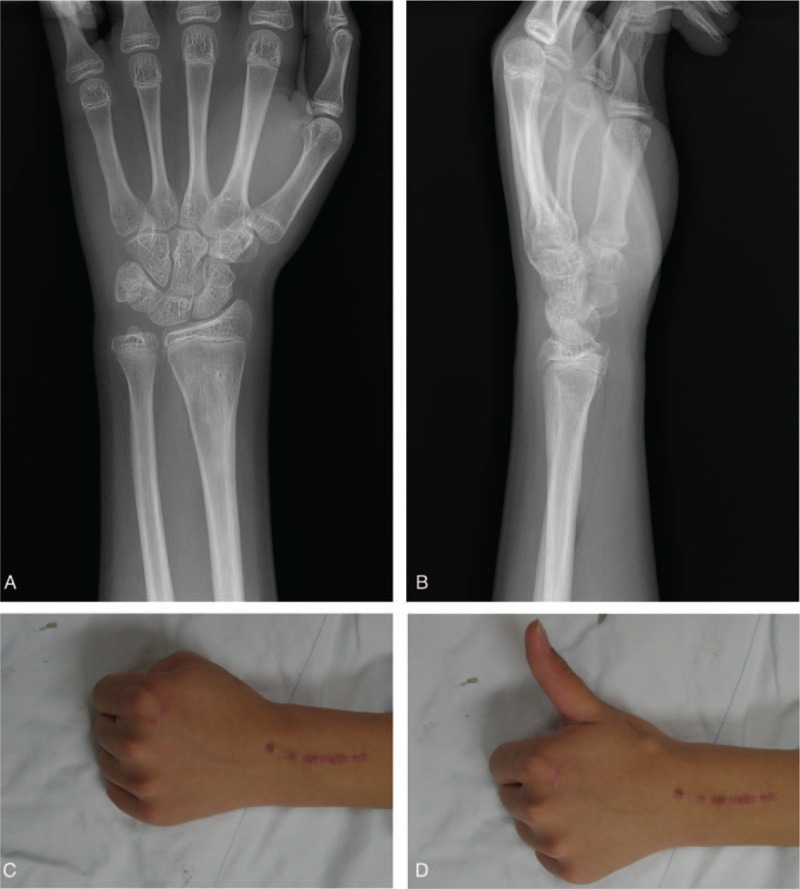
Plain radiographs (A, B) and photographs (C, D) obtained 10 months after the initial injury showing complete union of the fracture and full flexion and extension of the thumb.
